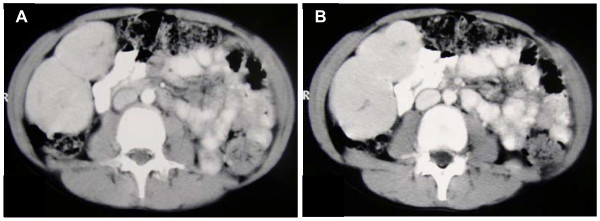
Abdomen CT scan showed two masses, with an evidence of tumor capsule, that, after contrast injection, were hyperdense in early (arterial) phase (A) and isodense, compared to the remaining liver parenchyma, in venous phase (B); it also highlighted a central hypodense "scar".
